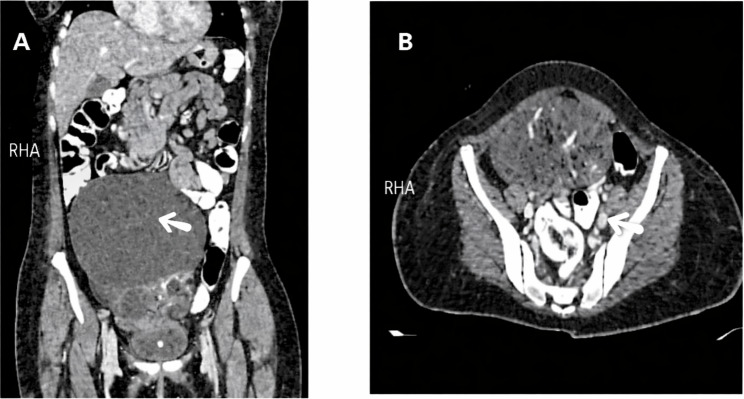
CT scan with IV contrast, image (A) showing coronal view, Image (B) showing cross sectional view (pre-op) showed solid and cystic and components of patient teratoma
